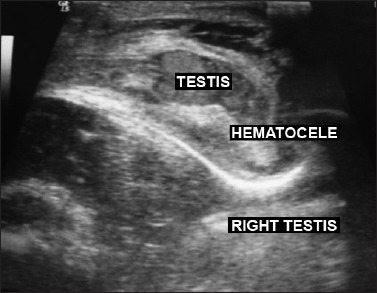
Scrotal ultrasonography showing blood accumulation in the right testis, consistent with hematocele.
